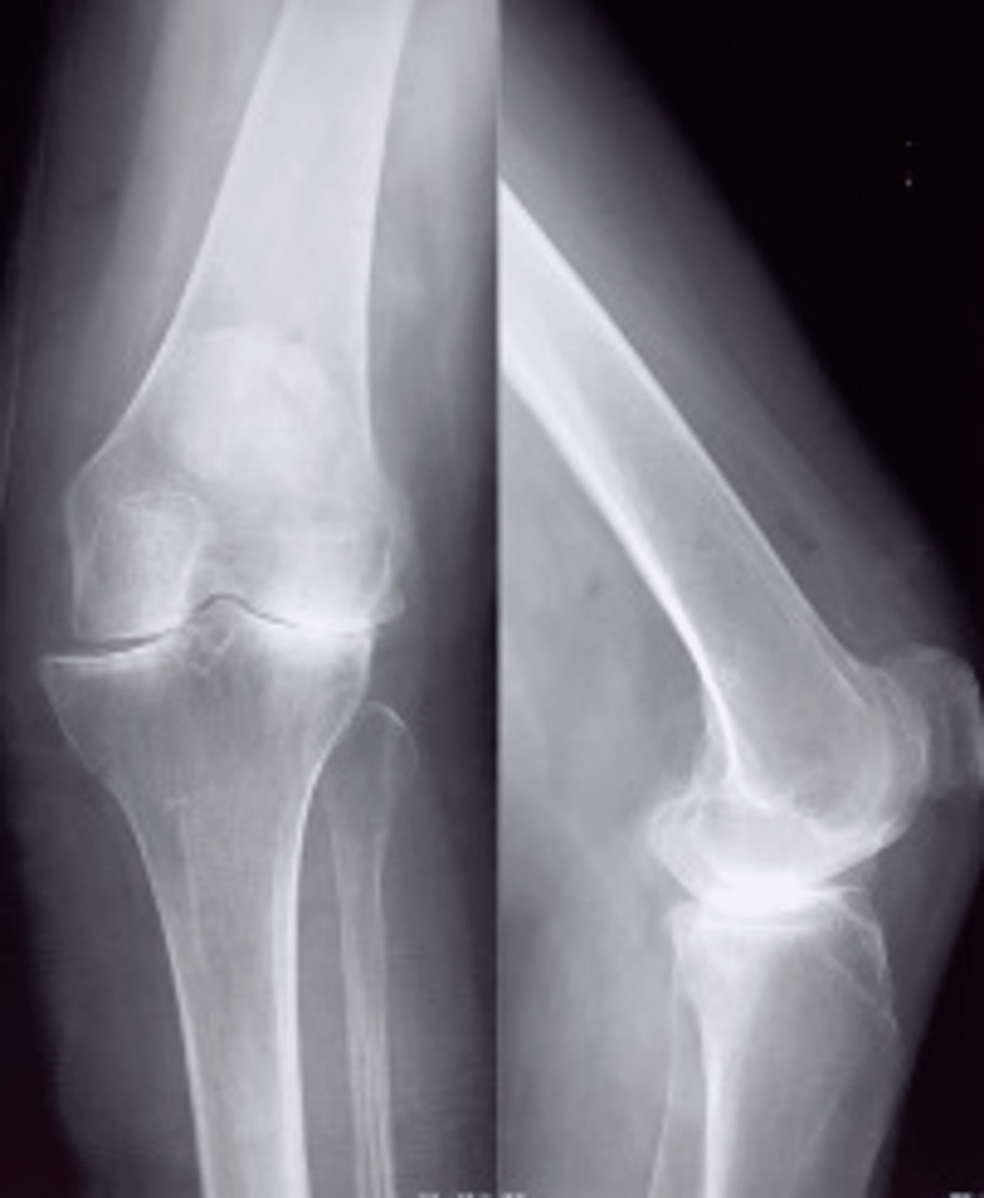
X-ray images of the affected knee Stage IV left gonarthrosis (Kellgren and Lawrence classification) previous to the Total Knee Arthroplasty
